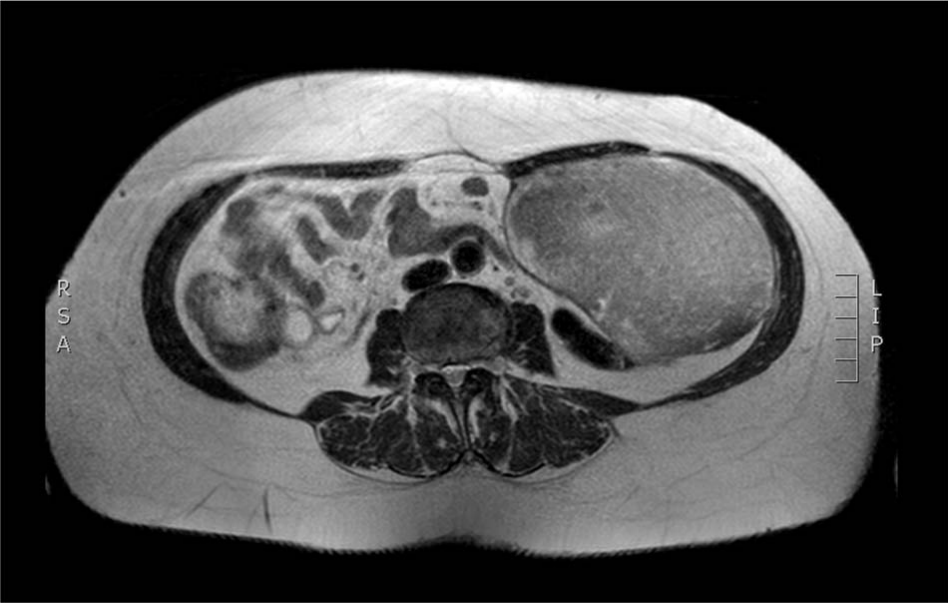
Preoperative MRI. T2 weighted image. Low sign density of the lesion.
